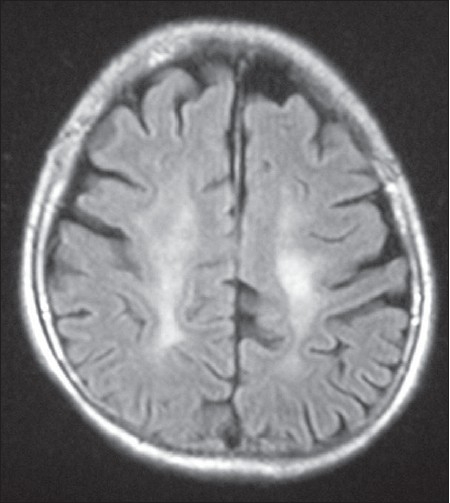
Axial FLAIR image showing white matter lesions in centrum semiovale parallel to interhemispheric fissure with frontoparietal lobe atrophy
